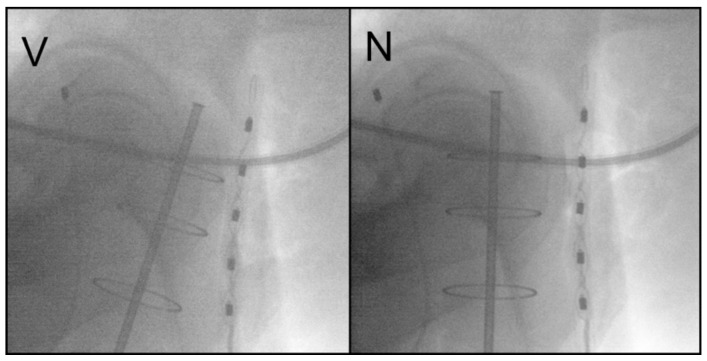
Lateral pre-interventional X-ray, before (V) and after (N) correction of the applicator position with consecutive reduction in the applied rectal dose.
